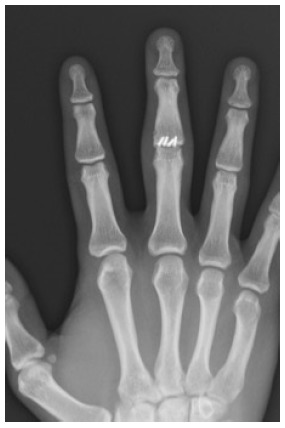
Patient 5 AP radiograph at final follow-up
